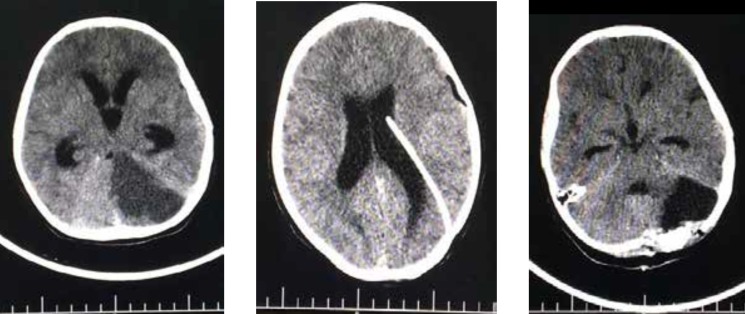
Brain CT scan on fourth day showing infarction on the same area along with obstructive hydrocephaly (left), Brain CT scan after inserting the drain in left lateral ventricle (middle), Brain CT scan after hemispherectomy of the left hemisphere of the cerebellum and extracting the drain (right).
